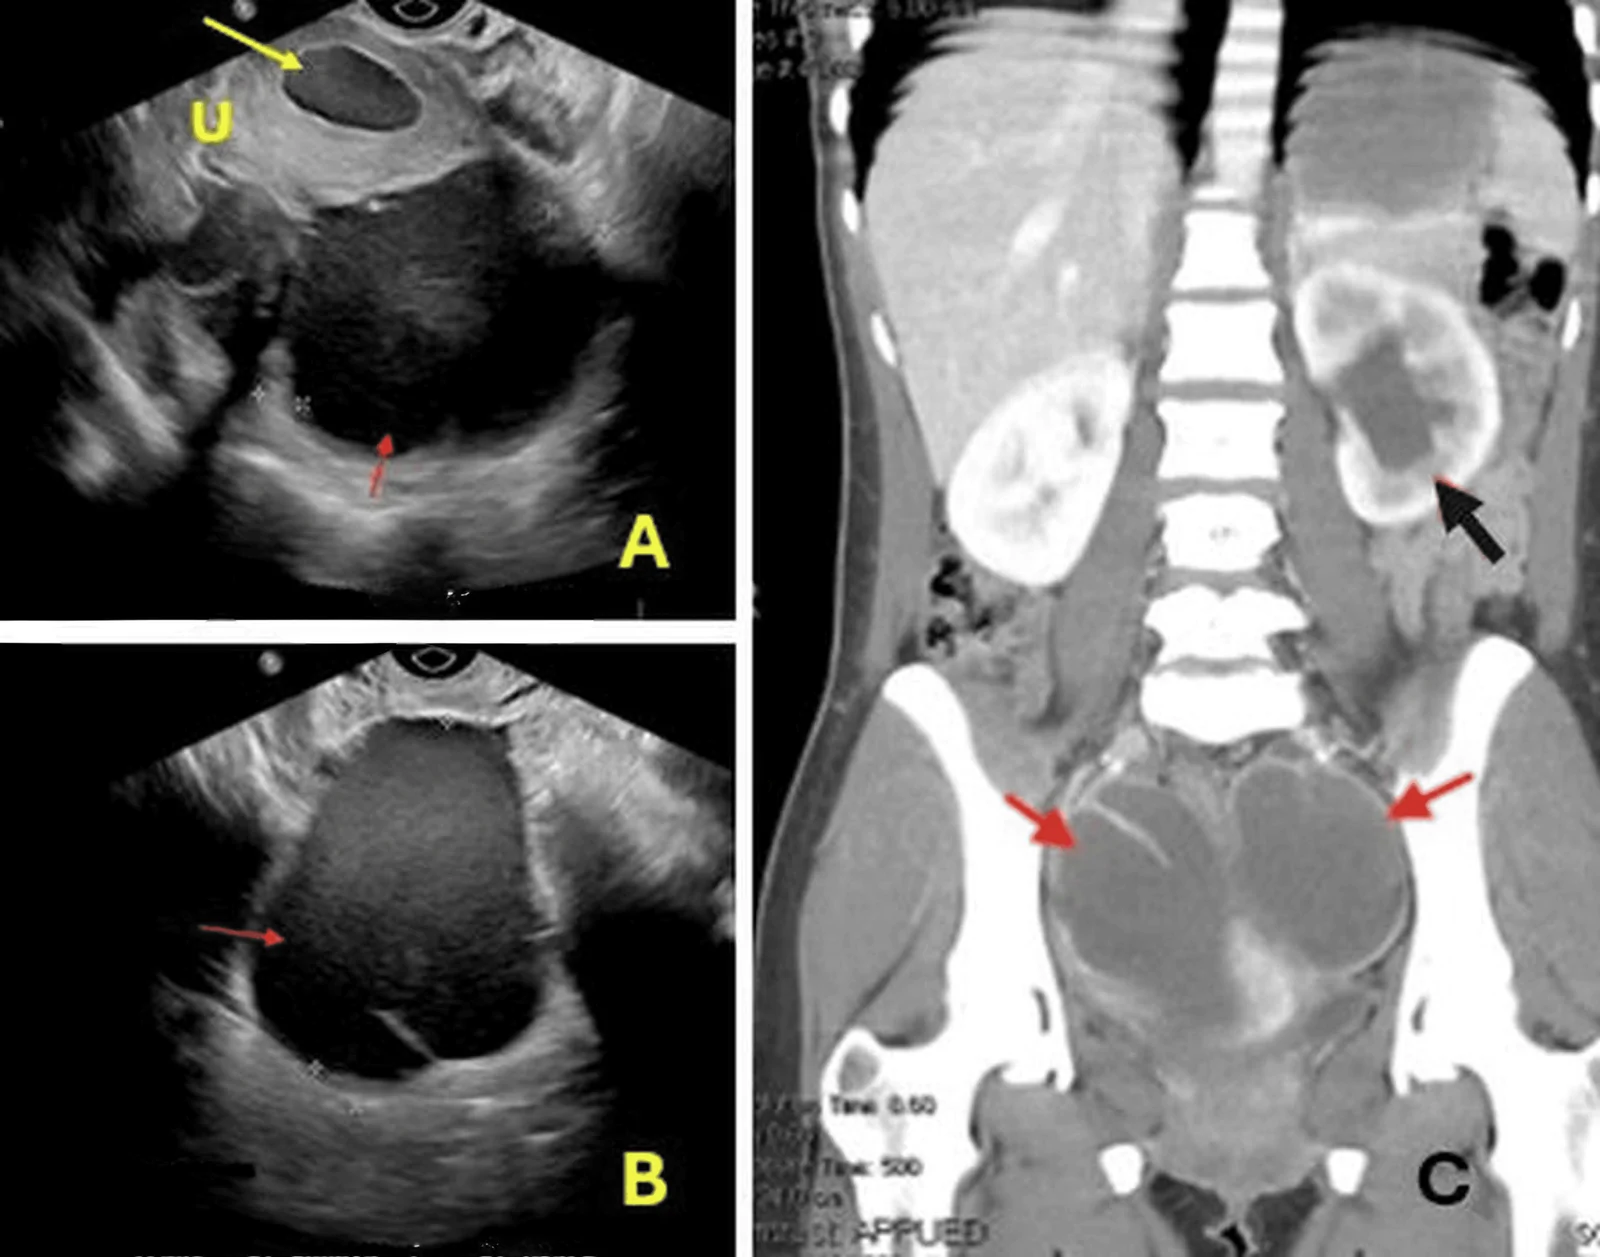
Follow-up pelvic imaging demonstrating progressive hematosalpinx and persistent left hydronephrosis "A" shows the left adnexa, and "B" shows the right adnexa. "U" indicates the uterus, and the yellow arrow indicates the fluid-filled endometrial canal. The red arrows indicate the pelvic masses. There is an interval increase in the volume of bilateral hematosalpinx, greater on the right than on the left, as well as increased hematometra. The left ovarian volume is 258.3 mL, compared with 224 mL previously, while the right ovarian volume is 694.7 mL, compared with 284 mL previously. "C" is a contrast-enhanced CT image of the abdomen and pelvis demonstrating persistent left hydronephrosis (black arrow) and hydroureter without an obstructing distal calculus. Bilateral complex, septated adnexal cystic lesions appear unchanged (red arrows), consistent with previously documented hydrosalpinx. TVUS: transvaginal ultrasound, CT: computed tomography
